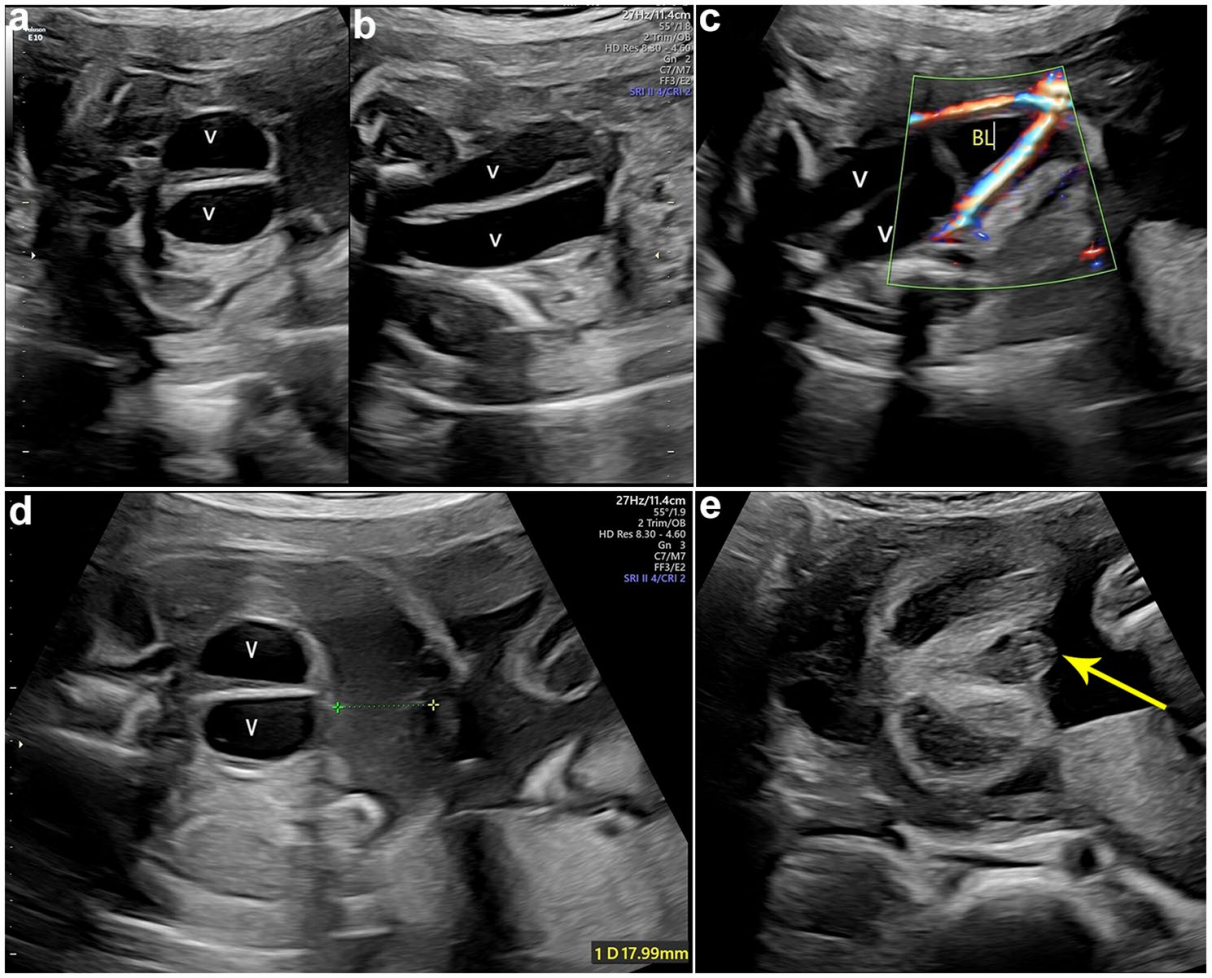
Ultrasound image at 27 weeks of gestation. Transverse (a) and longitudinal (b) views of the fetal pelvis show a centrally septated tubular structure, indicating duplicated and fluid-filled vaginal canals (V). (c) Centrally septated tubular structure behind the bladder (BL) that represents duplicated dilated vaginal canals(V). Doppler is used to delineate bladder position. (d) Transverse views of the fetal pelvis, showing duplicated vaginal canals (V) and fetal ascites (cursors). (e) Transverse sonogram of perineum hypertrophy of clitoris (arrow)
